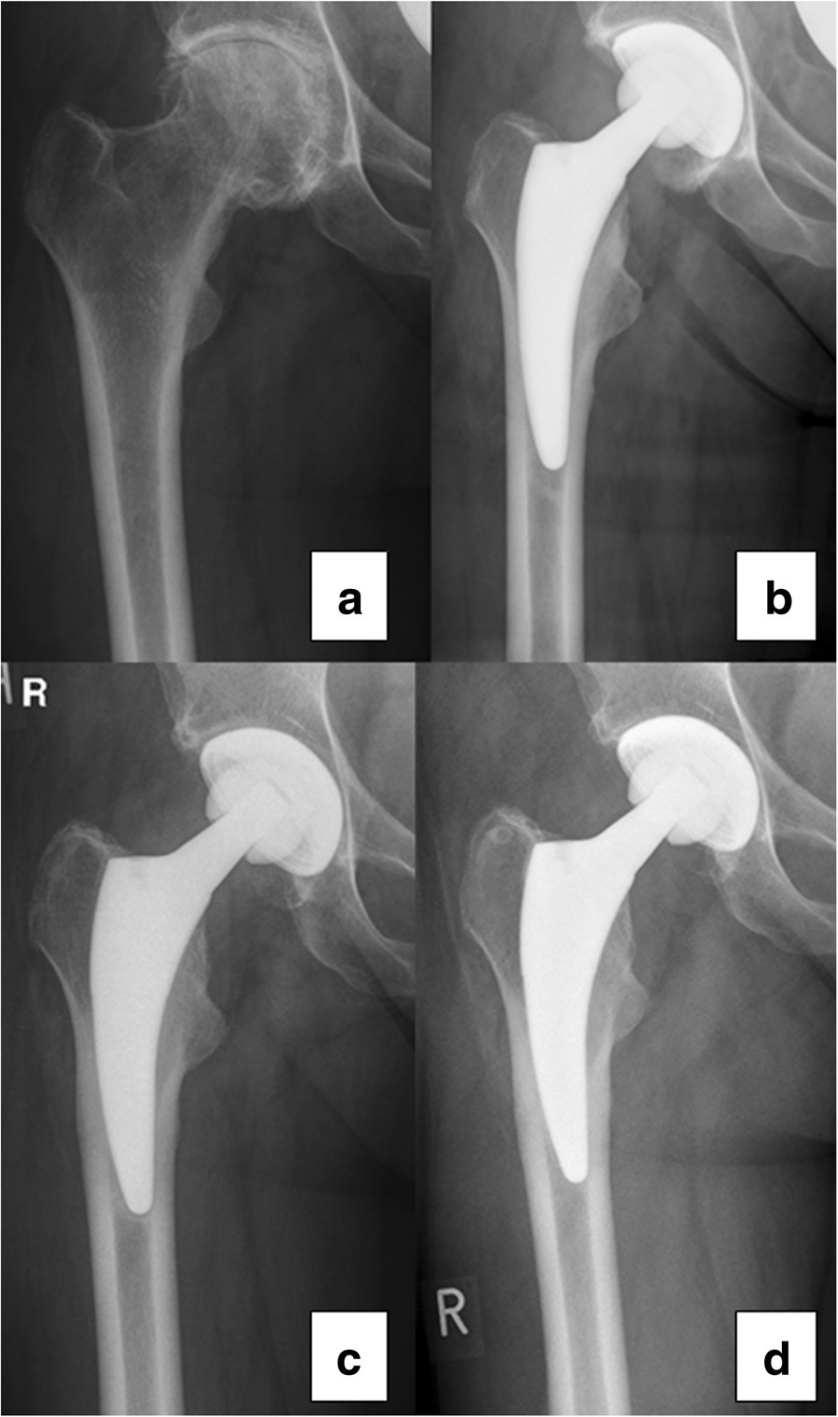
Anteroposterior hip radiographs of a 65-year-old woman with primary OA (a), directly postoperatively (b), 1 year (c), and 4 years (d) after surgery. The postoperative follow-up ((c) and (d)) shows the typical cortical hypertrophy mainly located in Gruen zone 3 and 5 and radiolucency < 2 mm in Gruen zone 1
